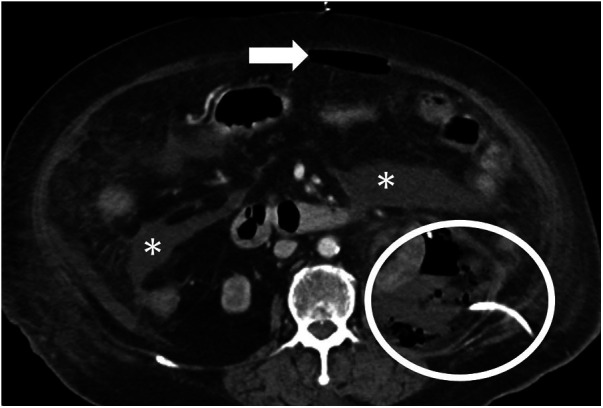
Abdominal CT findings suggestive of an intra-abdominal abscess related to an infected renal hematoma. The image reveals preexisting renal hematoma/abscess with pigtail (circle), pneumoperitoneum (arrow), and fluid collection, consistent with an abscess (asterisk).
